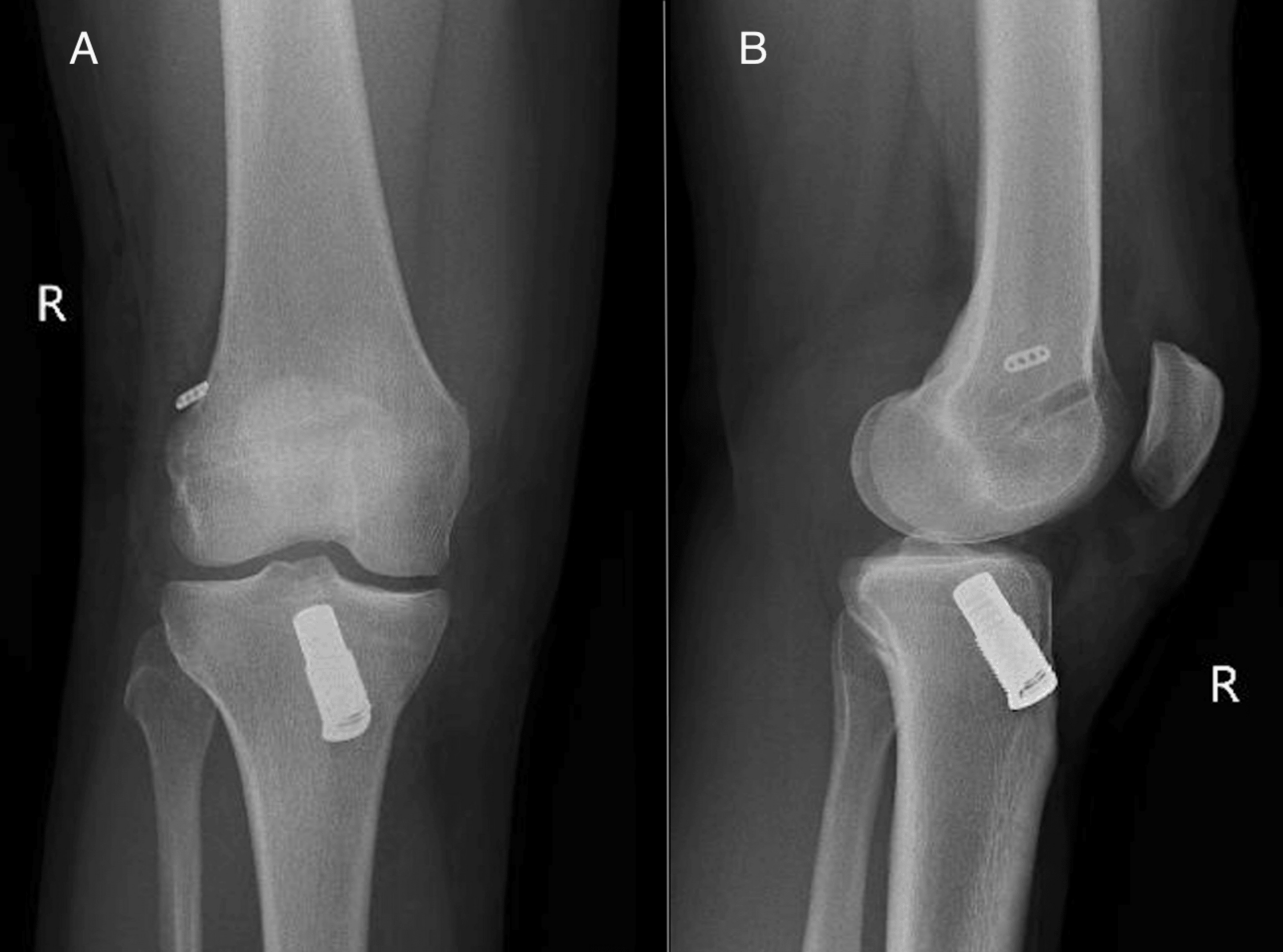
Postoperative anteroposterior (A) and lateral (B) radiographs of the right knee.
